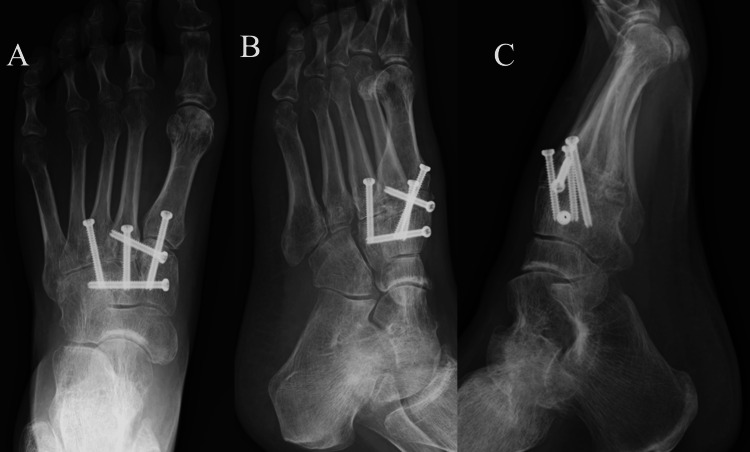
Radiographs of the left foot at six months postoperatively (A) Anteroposterior view. (B) Medial oblique view. (C) Lateral view. Anatomic alignment of the tarsometatarsal joints is maintained, with no evidence of post-traumatic arthritic changes.
